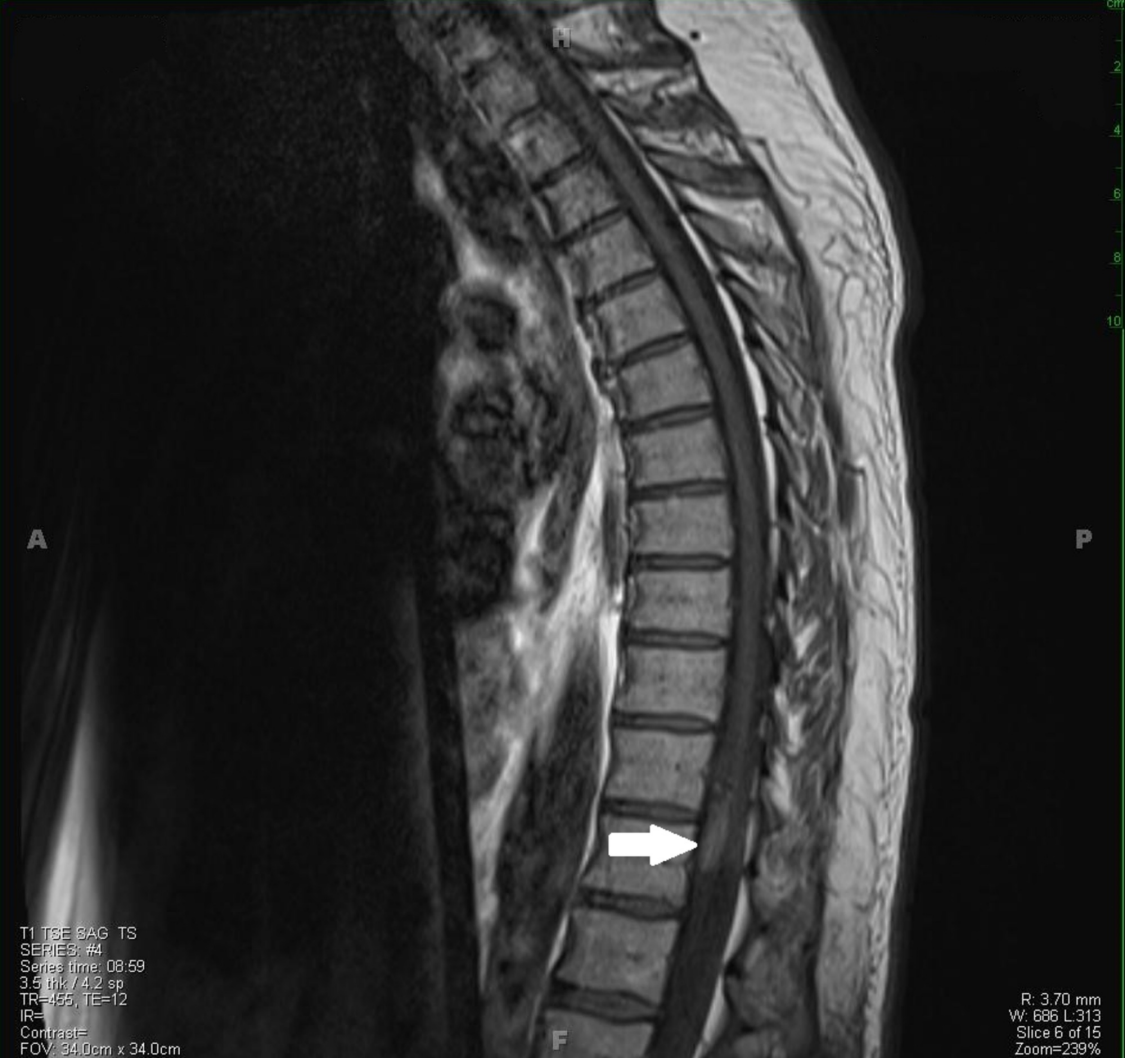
T1-weighted sagittal MRI displaying the intradural lesion.
